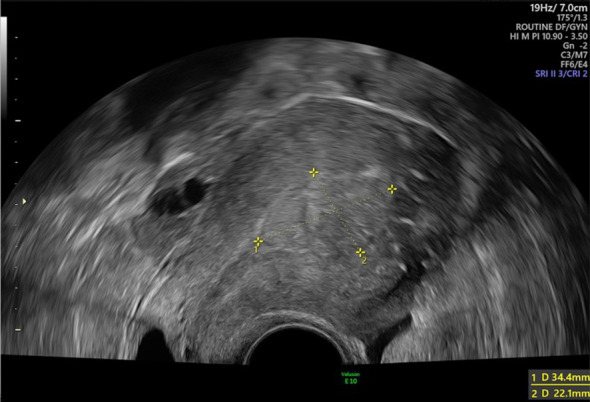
Image captured at the time of ultrasonographic staging of the tumor, displaying uterine cavity, residual lesion, and visible suture in the uterine fundus after previous surgery.
